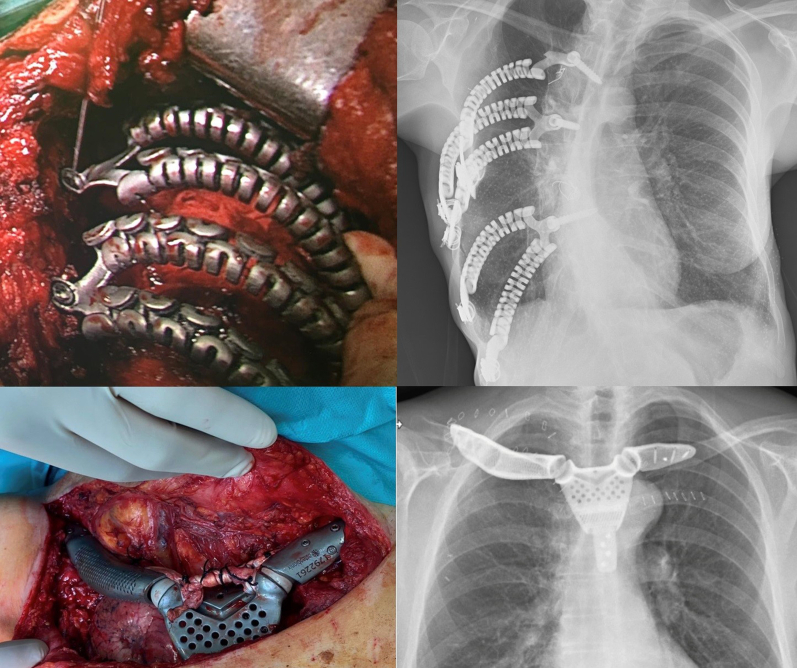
Intraoperative image of costovertebral and sternoclavicular prosthesis and postoperative radiograph.
